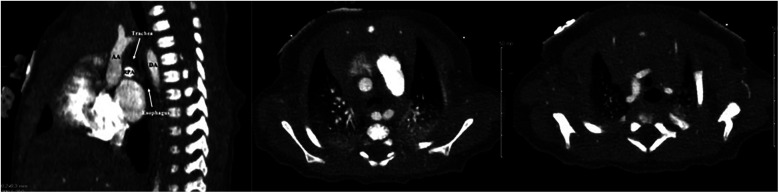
Cardiac computed tomography (CT) images demonstrating sagittal and axial views of the esophageal and tracheal compression caused by the vascular ring (middle panel taken at the level of the aortic arch for comparison to the right panel taken above the level of the aortic arch). AA, ascending aorta; DA, descending aorta; RPA, right pulmonary artery.
